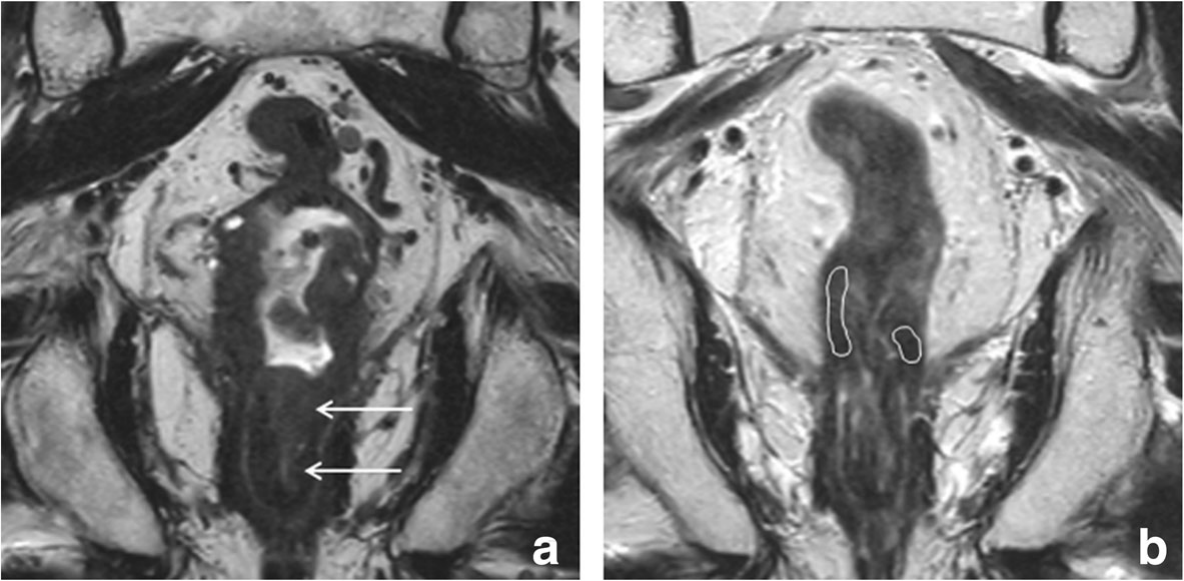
Assessment of sphincter involvement. Coronal T2-weighted MR images of a male patient with a tumor in the distal rectum before (a) and after CRT (b). Before treatment the tumor extends into the proximal one third of the anal canal where it invades the internal anal sphincter on the left lateral side (arrow in a). After CRT, the tumor has decreased considerably in size and retracted from the anal canal. A fibrotic remnant (circles in b) remains visible above the level of the anorectal junction. The anal sphincter is no longer involved
